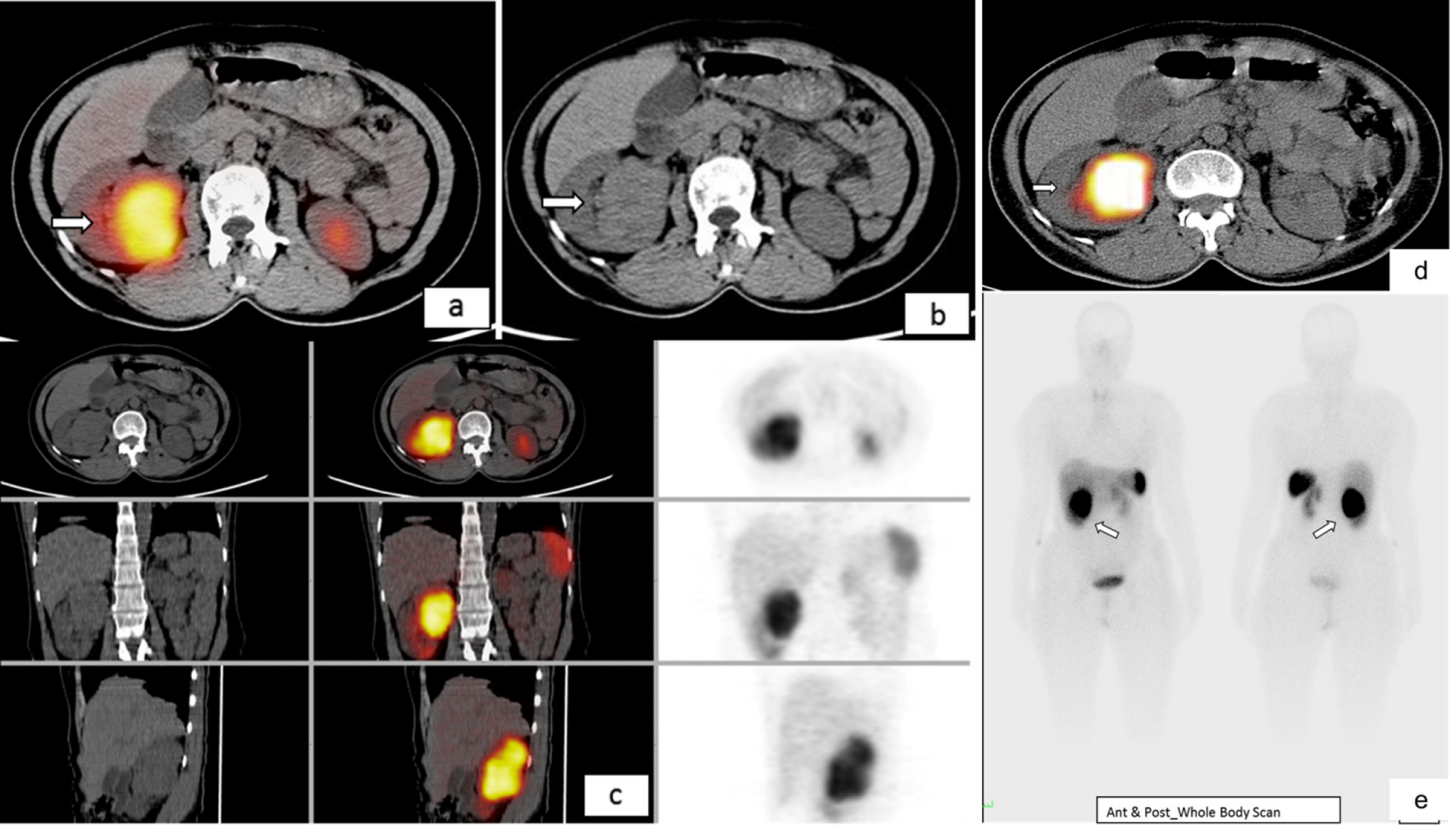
Nuclear scans. 3 mCi of 68Ga-Dotanoc PET/CT scan demonstrates somatostatin receptor (SSTR) expressing an intrarenal mass (2a) that corresponds to NCCT mass (2b). There was no abnormal SSTR expressing tumour in pancreas (2c), or any other abdominal structure. Similar observation was initially made from 15 mCi of 99mTc-HYNICTOC SPECT/CT scanning (2d, 2e).
